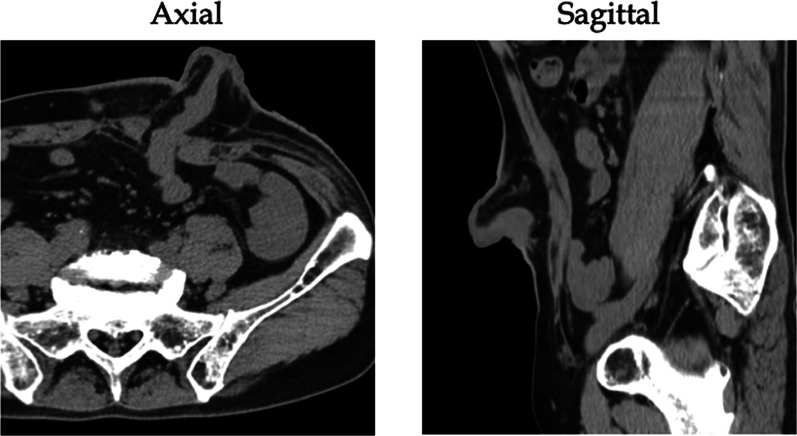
Abdominal computed tomography. Computed tomography of the abdomen showing no evidence of intra-abdominal abscess or inflammation around the stoma
